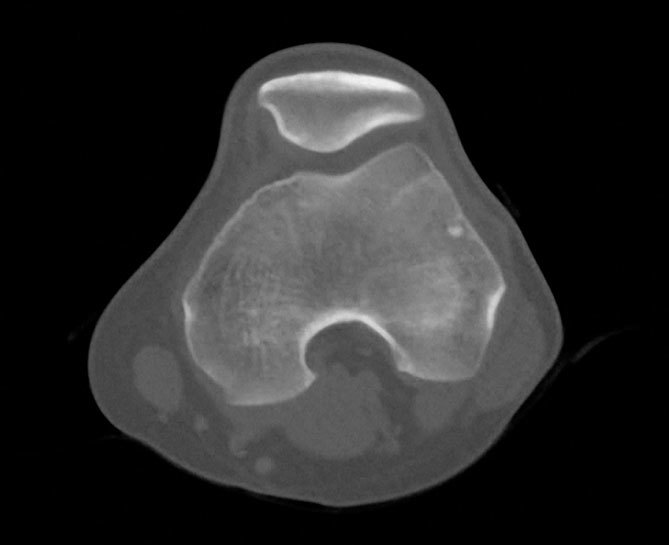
CT image of the knee shows the osteoid osteoma with a very small and calcified nidus.
